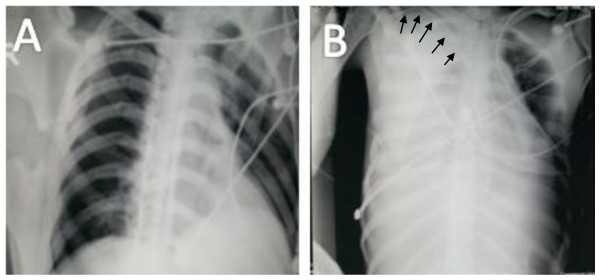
Revealing a normal anteroposterior chest radiograph before subclavian catheterization (panel A). chest X-ray performed after catheterization revealing massive left sided pleural effusion with mediastinal shift to the left side. Arrows point to the course of the catheter.
